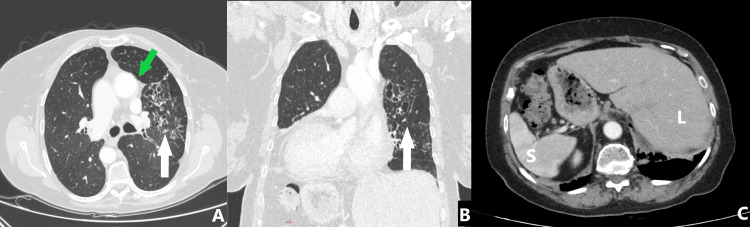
Chest CT. Chest CT (with intravenous contrast) showing (A) cystic bronchiectases mostly on the left upper lobe and lingula and diffuse mosaic attenuation (white arrows). The ascending aorta is located on the left (green arrow). No other cardiac anomalies are noted (not shown). (B) Dextrocardia and bronchiectasis (white arrows) on the left upper lobe. (C) Situs inversus. The liver (L) and spleen (S) are reversed.
